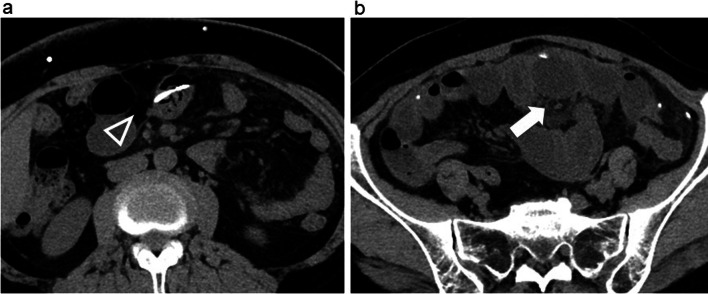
a CT scan showing that the tip of the right shunt catheter had entered the lumen of the small intestine (white arrowhead). b CT scan showing abscess formation on the caudal side of the perforating catheter in the small intestine (white arrow)
